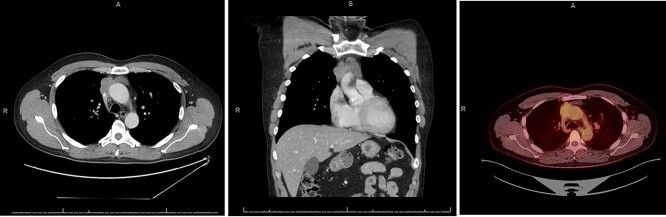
CT chest axial (left), coronal (middle) and PET (right) of invasive thymoma.
